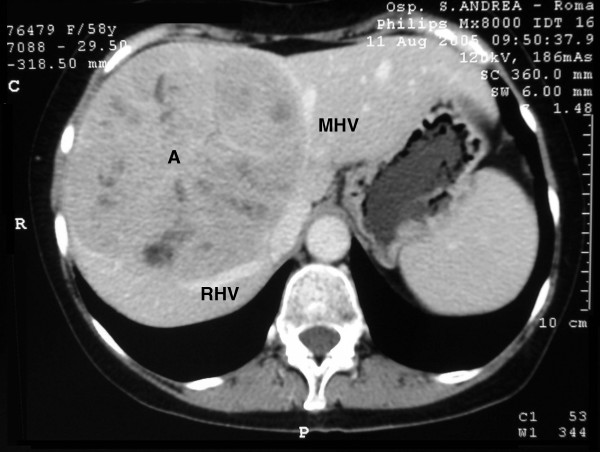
Contrast-enhanced CT scan. A: adenoma; Cy: cyst; C: calcifications within the cystic lesion (arterial phase);.
